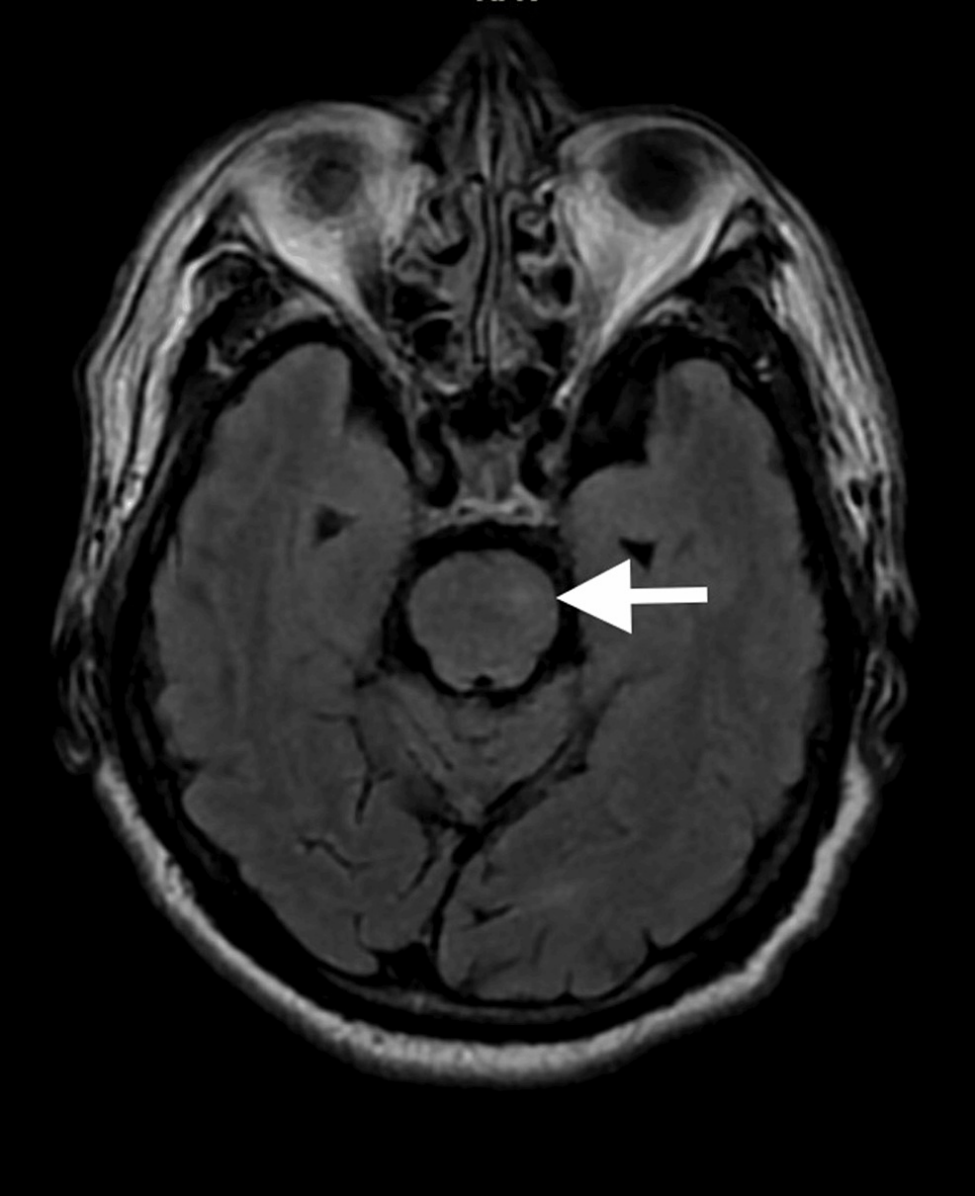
MRI brain showed FLAIR hyperintense lesion (arrow) at the level of left pons MRI, magnetic resonance imaging; FLAIR, fluid-attenuated inversion recovery
